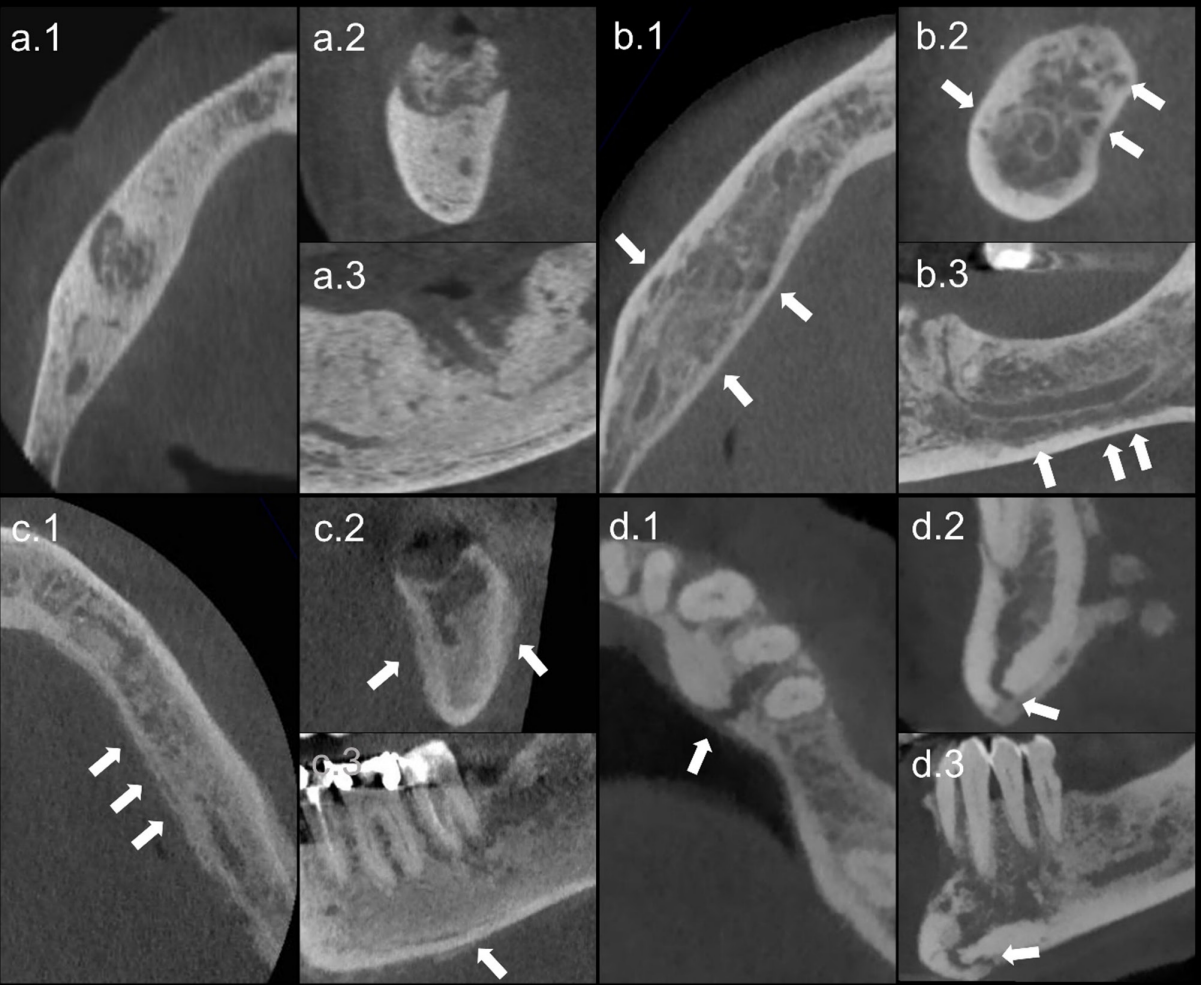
Cropped CBCT reconstructions exampling the imaging features associated with MRONJ in (1) axial, (2) coronal, and (3) sagittal slides of CBCTs. (a) Deep and extensive osteosclerosis with a superficial and localized bone sequestrum. (b) Deep and extensive osteolytic lesions pointed out with white arrows. (c) Extensive periosteal reaction. (d) Mandibular fracture. CBCT, cone beam CT; MRONJ, medication-related osteonecrosis of the jaws.
